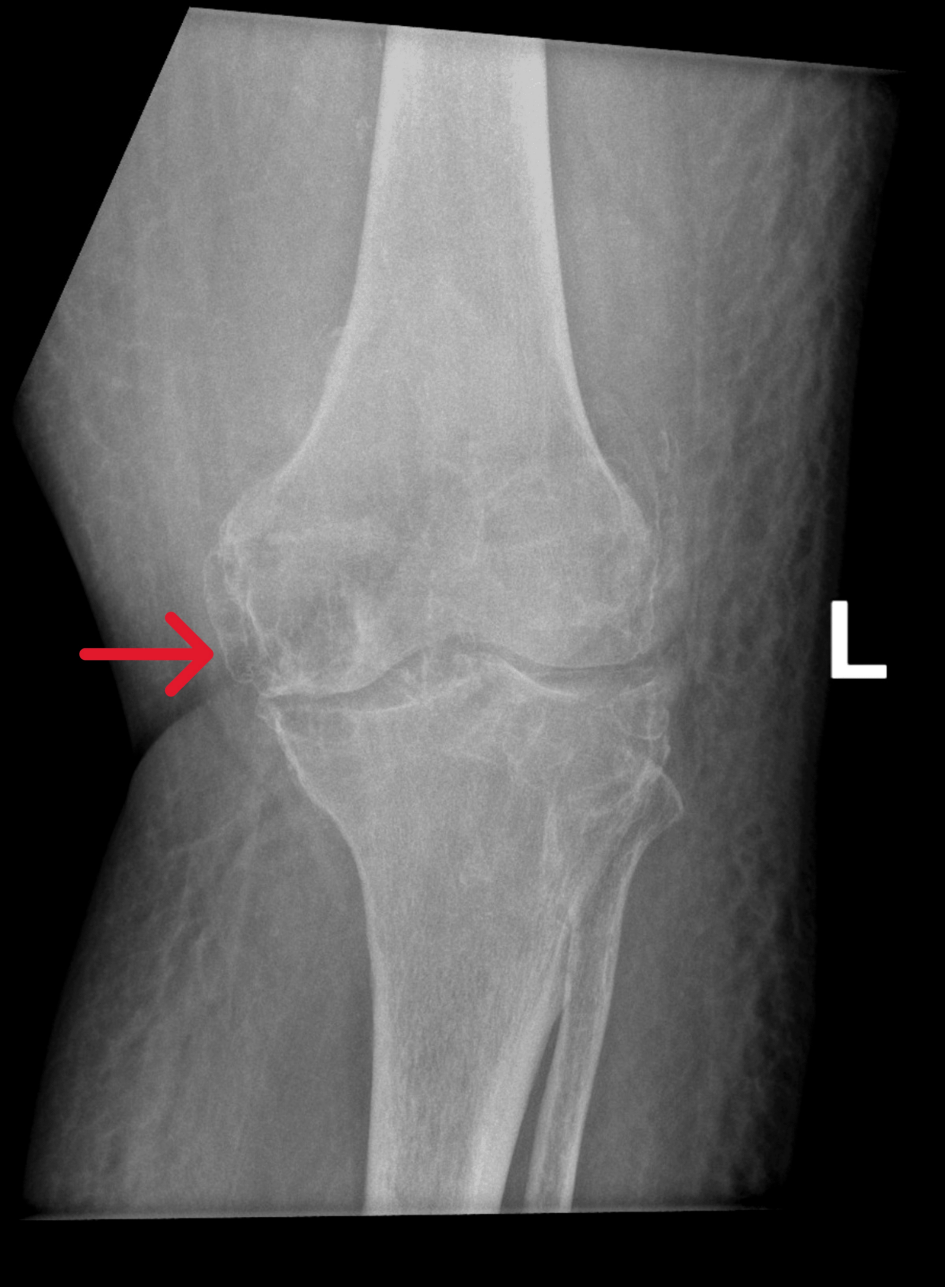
An X-ray (AP view) demonstrates subarticular radiolucencies in the medial aspect of the distal femur AP view: anterior-posterior view
